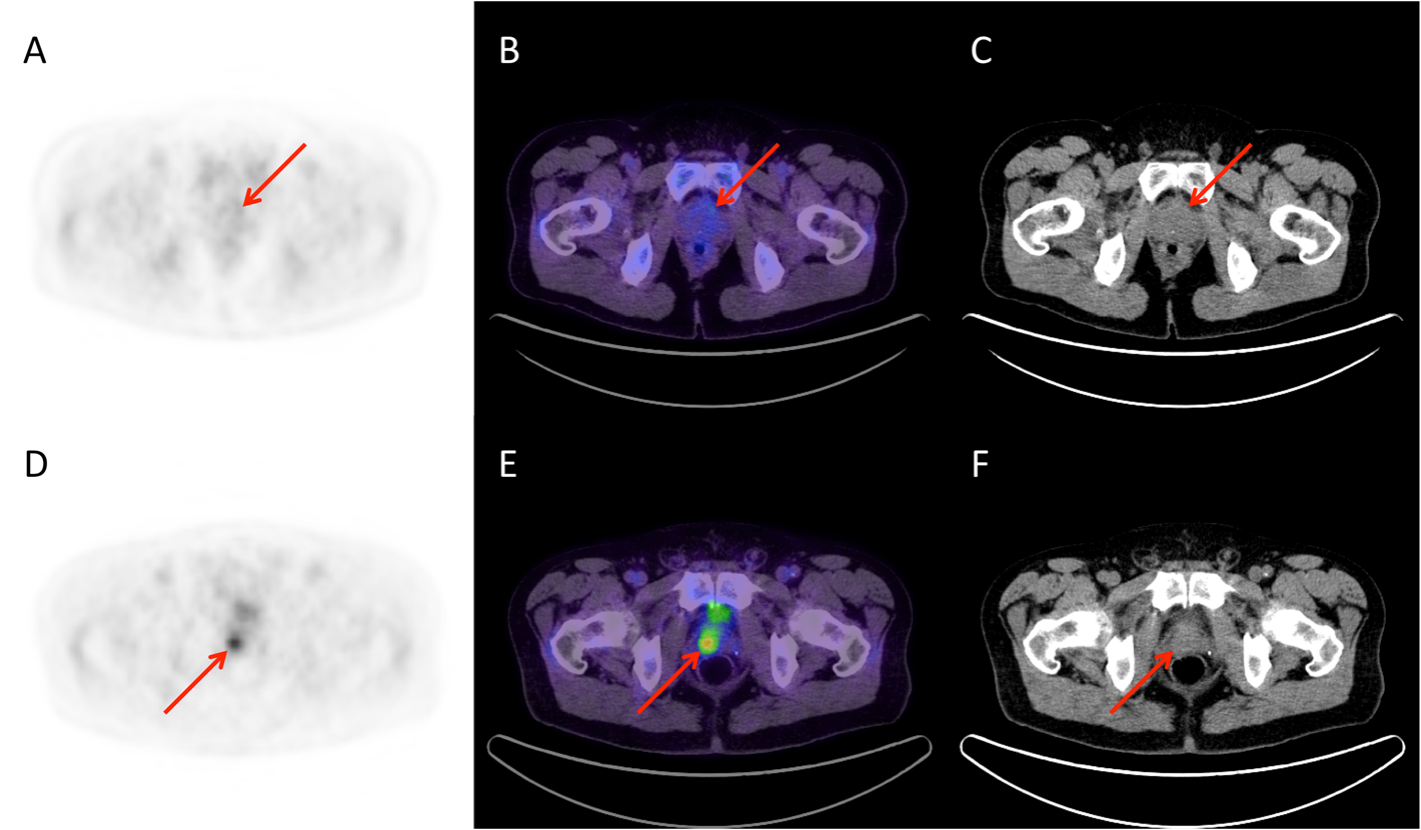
Examples of corresponding transaxial PET (A, D), fused PET/CT (B, E) and CT (C, F) slices. A patient (A-C) had a Gleason sum 8 (4 + 4) PCa at biopsy and the most prominent focus of prostatic FDG uptake was only faintly noticeable (negative clinical report; Score = 1; SUV_max_ = 2.7). His PCa was downgraded to Gleason sum 6 (3 + 3) after RP. Conversely, another patient (D-F) also had a Gleason sum 8 (4 + 4) PCa at biopsy, but FDG-PET/CT showed a highly hypermetabolic prostatic focus (positive clinical report; Score = 3; SUV_max_ = 8.2). His PCa was upgraded to Gleason sum 9 (5 + 4) following RP.
